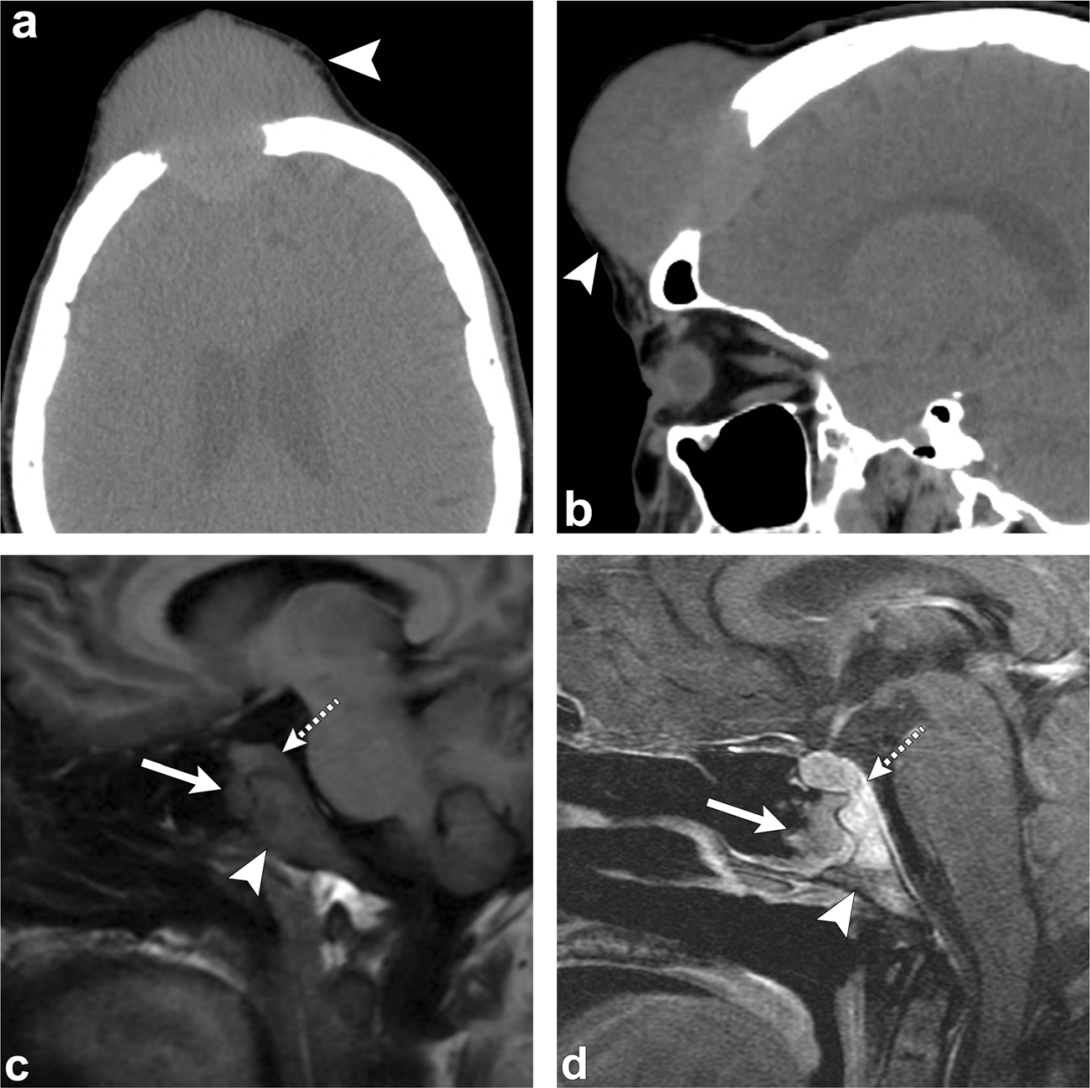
Skull metastasis. *Patient 1:* Axial (a) and sagittal (b) head CT images show an expansile destructive lesion in the frontal skull (*arrowheads*), pathology proven metastatic renal cell carcinoma. *Patient 2:* Sagittal T1-weighted (c) and sagittal post-contrast T1-weighted (d) images demonstrate an enhancing lesion in the clivus (*arrowheads*) with soft tissue component extending into the sphenoid sinus (*arrows*) and anterior to the midbrain (*dashed arrow*). This lesion was proven to be metastatic from breast primary
